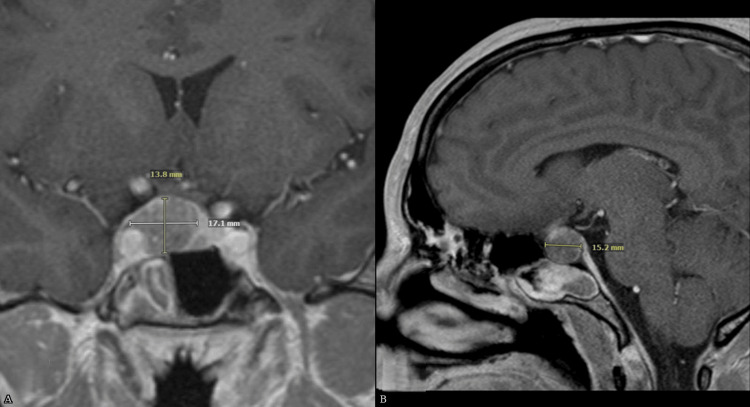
Pituitary magnetic resonance imaging (MRI) demonstrating a thyrotropin (TSH)-secreting pituitary macroadenoma. (A) Coronal T1-weighted post-contrast MRI image showing a sellar mass measuring 17.1 × 13.8 mm centered in the right pituitary with upward displacement of the optic chiasm and prechiasmatic right optic nerve. (B) Sagittal T1-weighted post-contrast MRI image demonstrating superior extension of the macroadenoma measuring 15.2 mm in craniocaudal dimension.
